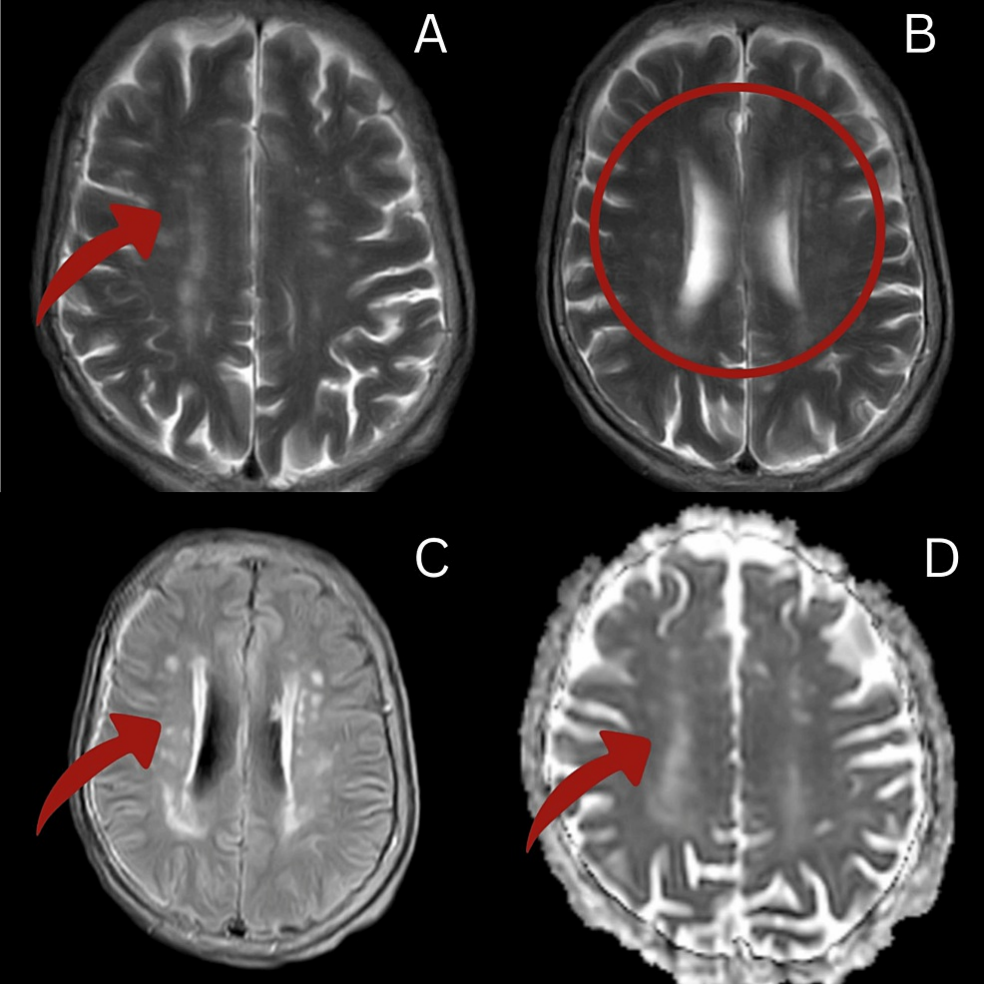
Different weighted images of MRI Brain A, B: eT2w TRA image, C: eAX FLAIR image, D: dADC image, Red arrow: showing bilateral extracapsular infarct MRI: Magnetic Resonance imaging
